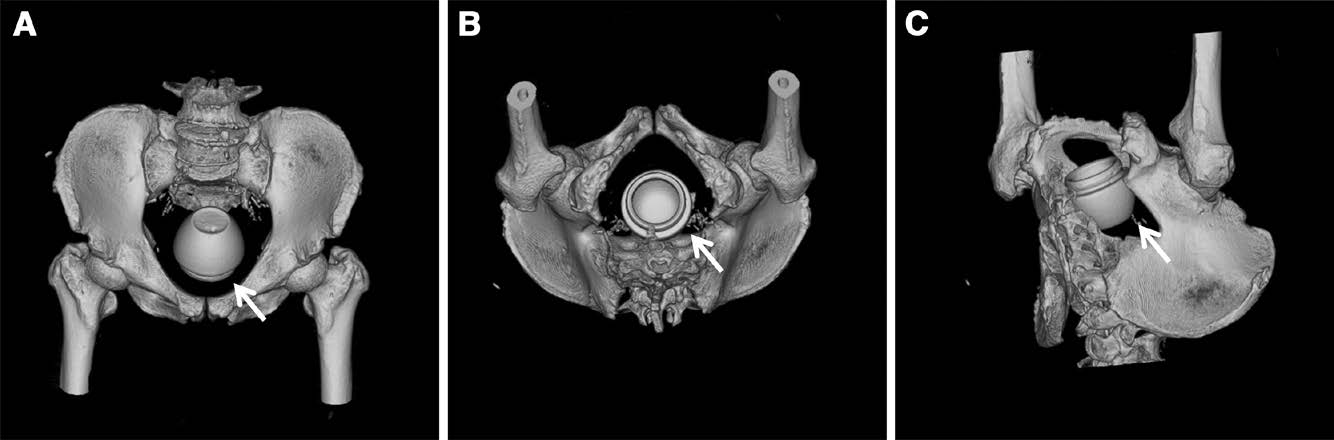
CT images (A–C) with the VRT show the 3D surface of the cup-shaped foreign body stuck in the rectal lumen (white arrow). 3D = three dimensional, CT = computed tomography, VRT = volume rendering technique.
